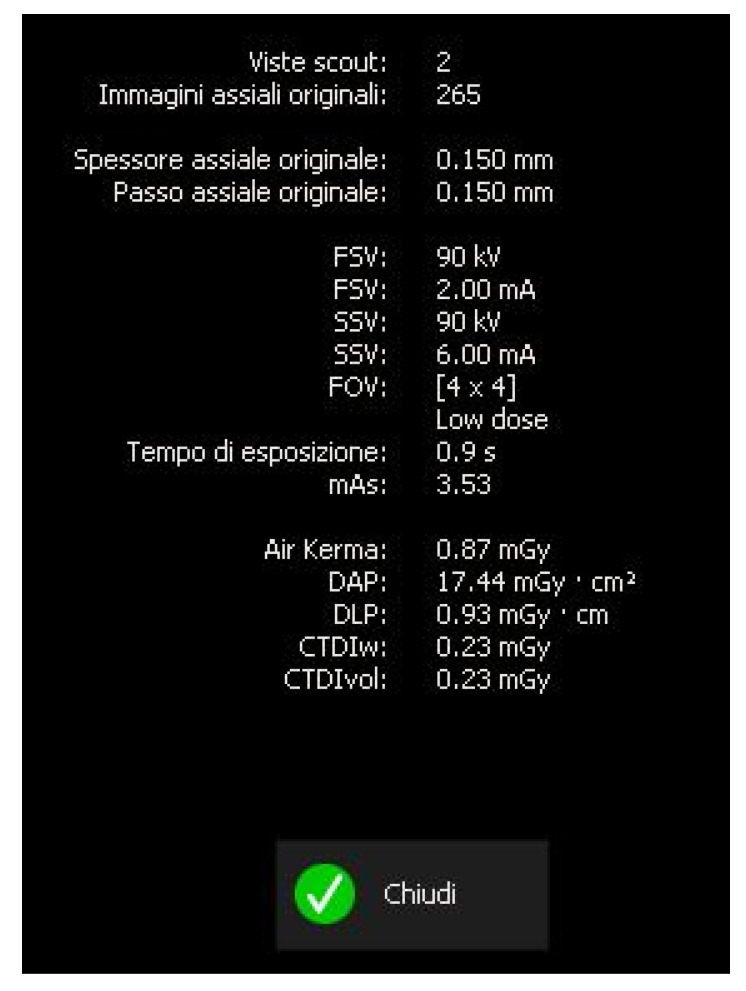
Exposure parameters of CBCT follow-up image.
